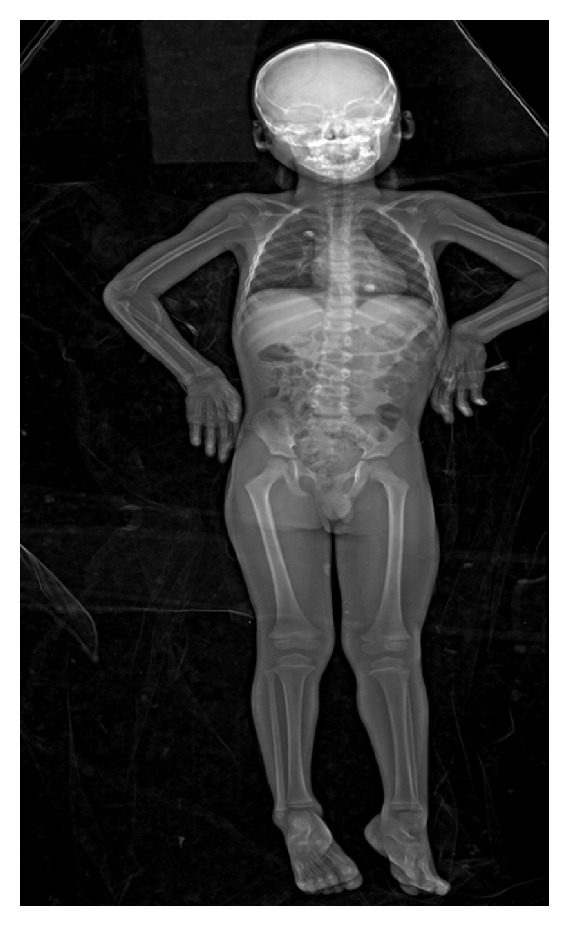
Paediatric full-body X-ray image showing superior imaging of the major airways.
